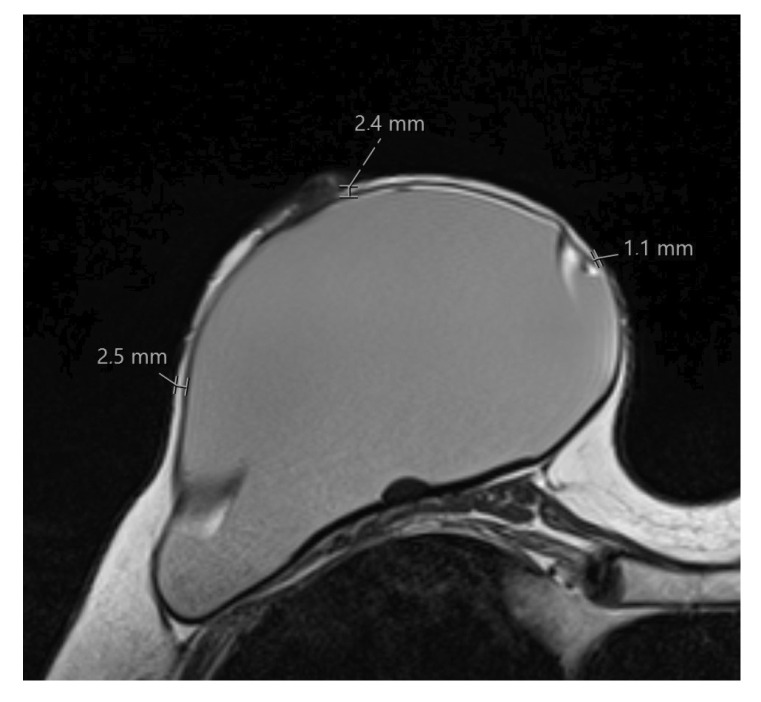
Skin flap measurement acquisition in T2-weighted image. Thickness measurements of retromamillary skin flap and at 3 and 9 o’clock. Measurements were performed using the integrated measurement tool of the Picture Archiving and Communication System (SECTRA AB, IDS7 Version 22.1, Linköping, Sweden).
